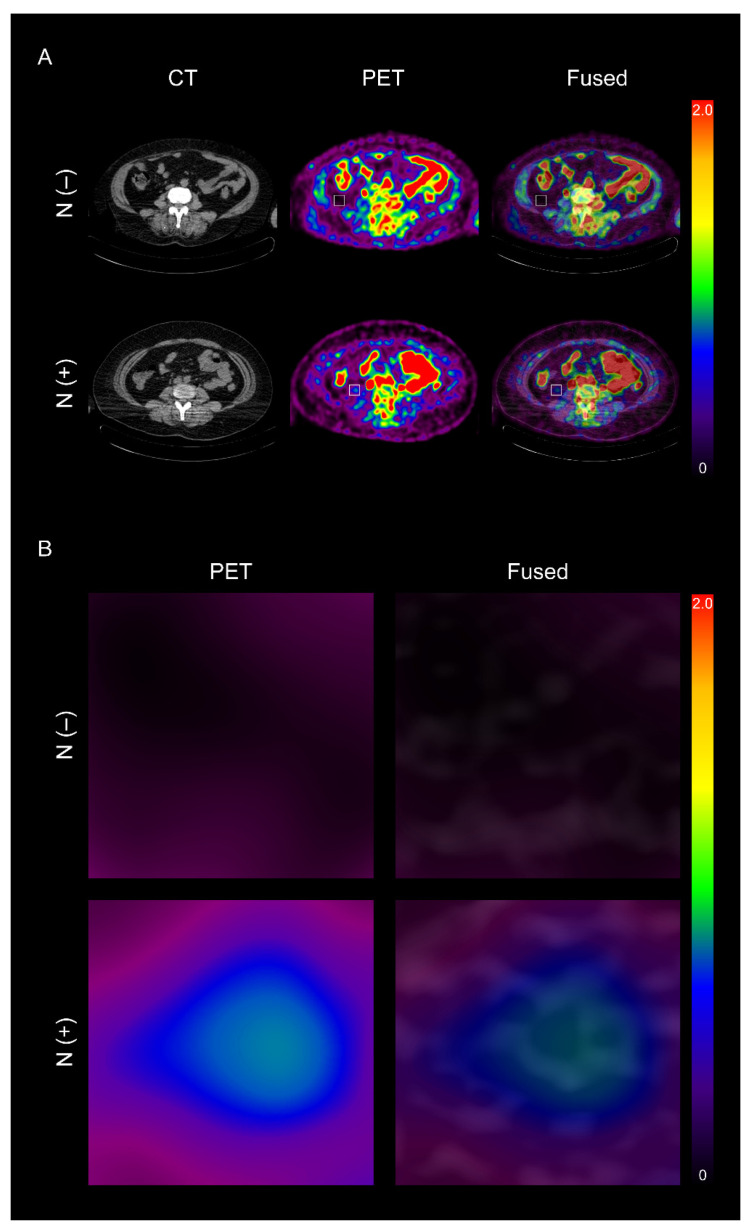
Representative ^18^F-FDG PET/CT images of visceral adipose tissue (VAT) metabolic activity according to lymph node (LN) metastasis status (A) and its corresponding magnified images of VAT (B). N (−): negative LN metastasis, N (+): positive LN metastasis, ^18^F-FDG: ^18^F-fluorodeoxyglucose, PET: positron emission tomography, CT: computed tomography.
